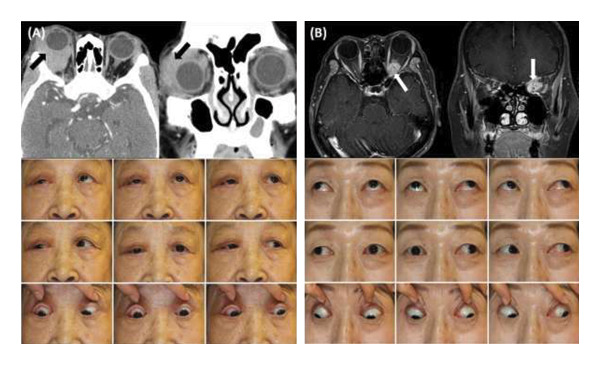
(A) Computed tomography (CT) showing lymphoma involving the right orbital cavity and lacrimal gland (black arrow). Nine gaze photos showing abduction limitation and ptosis in the right eye (case 1). (B) T1‐weighted magnetic resonance imaging (MRI) showing an optic nerve sheath meningioma in the left orbital apex (white arrow). Nine gaze photos showing adduction limitation in the left eye (case 19).
